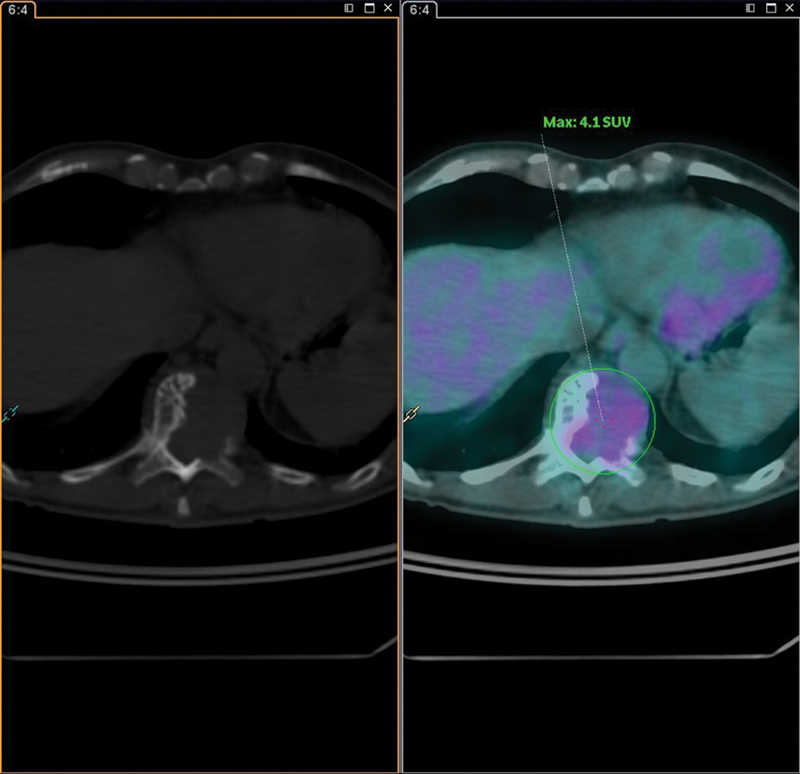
Axial computed tomography slices (
